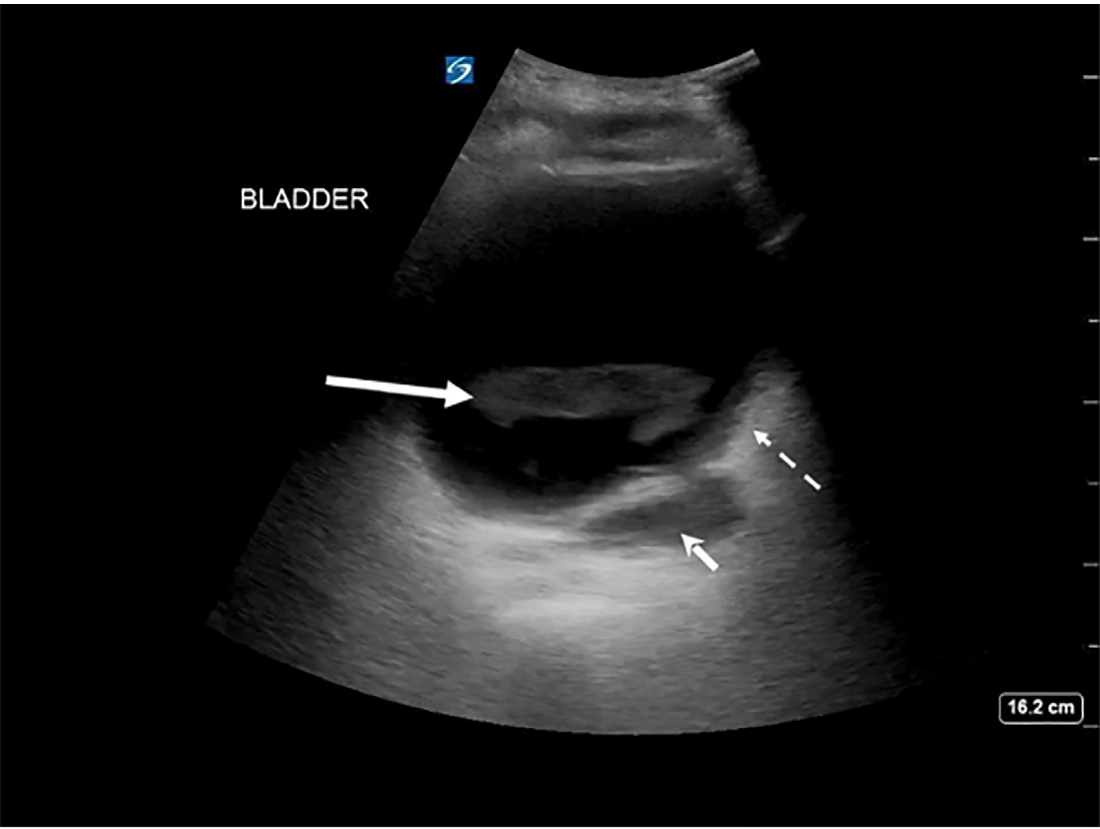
Transverse view of the bladder on point-of-care ultrasound showing echodense
